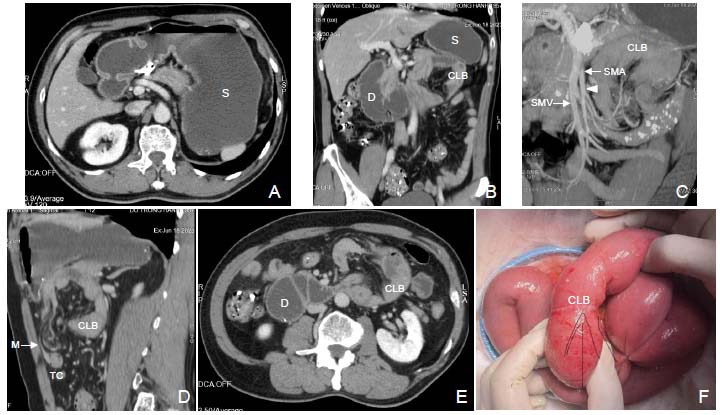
Multidetector-row computed tomography (MDCT) (A-E) and intraoperative (F) images of a 66-year-old male patient with dull epigastric pain. Axial (A, E) and coronal (B) CT images showing a dilated stomach (S) and duodenum (D) filled with fluid, suggesting a high intestinal obstruction. Maximum intensity projection (MIP) (C) and sagittal (D) CT images showing that the superior mesenteric artery (SMA) and vein (SMV) vascular bundle is pushed to the right (white arrowhead), and the transverse colon is pushed forward by the closed loop of the bowel (CLB). Sagittal (D) and axial (E) images show an inverted C-shaped first loop of the small intestine, herniated through the transverse colon mesentery, and located in the left-abdominal cavity superior-posterior to the transverse colon (TC) near the angle of Treitz. No signs of intestinal ischemia and no free fluid or air are apparent in the abdominal cavity. Intraoperative image (F) showing that the first loop of the small intestine was strangulated at the natural orifice in the transverse colon mesentery; however, the surface of the loop was still pink, with no signs of ischemia or necrosis. CLB = Closed loop of bowel, D = Duodenum, M = Transverse mesentery, S = Stomach, SMA = Superior mesenteric artery, SMV = Superior mesenteric vein, TC = Transverse colon.
